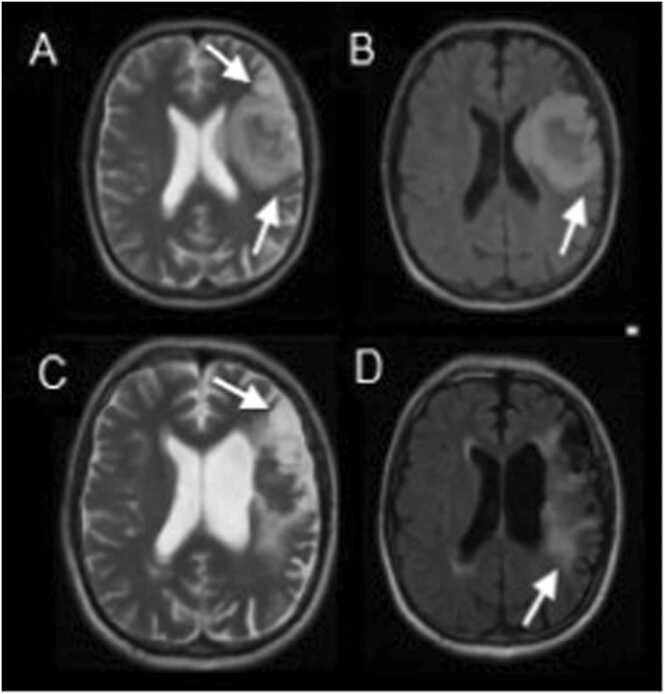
Axial sections of brain MRI. (A, C) Brain MRI at 8-months after heart transplant reveals increased signal intensity in the cerebral cortices with swelling on diffusion-weighted images and (B, D) T2-weighted images (arrows).
